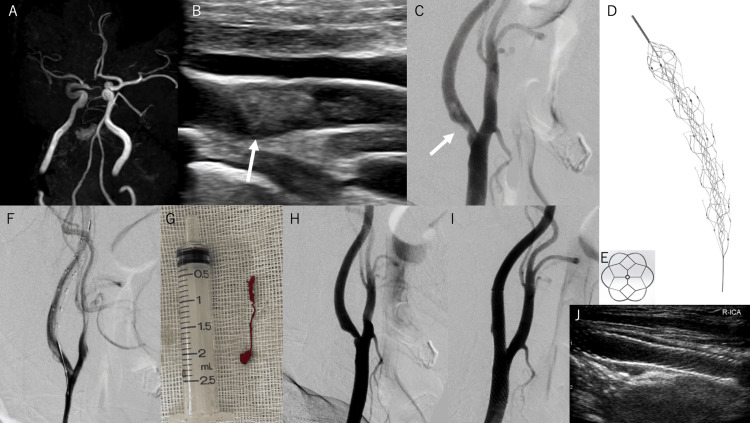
Treatment course of carotid artery occlusion A: The right distal ICA and middle cerebral artery cannot be visualized clearly on MRA. B: Longitudinal view of the right common carotid ultrasonography. An ulcerated plaque with FFT (white arrow), which moves synchronously with the heartbeat, is present beside the carotid plaque. C, F, H, I: Serial images from digital subtraction angiography. Carotid artery stenosis and FFT (C). Embotrap III (5×37 mm) was deployed in the distal ICA (F). After aspiration, the FFT was removed (H). After Wallstent deployment, the carotid lumen was dilated to a normal diameter (I). White arrows indicate FFT. D: Embotrap III. E: Distal basket of Embotrap III (front view). G: A string-like thrombus collected by aspiration. J: Follow-up carotid ultrasonography on day 1. Stent stenosis was not observed ICA: internal carotid artery; MRA: magnetic resonance angiography; FFT: free-floating thrombus
